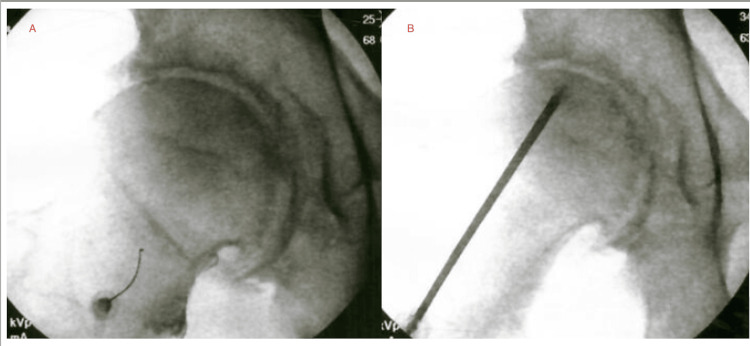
Patient 2 - right hip BMC injections Fluoroscopic-guided intra-articular (A) and intraosseous (B) BMC injections in a 65-year-old male (Tönnis 2). MRI revealed cartilage thinning and osteophyte formation. Patient 2 reported VAS improved from 7 to 2, and at least 50% SANE score improvement in function. BMC, bone marrow concentrate; VAS, visual analog scale; SANE, single assessment numeric evaluation; MRI, magnetic resonance imaging
